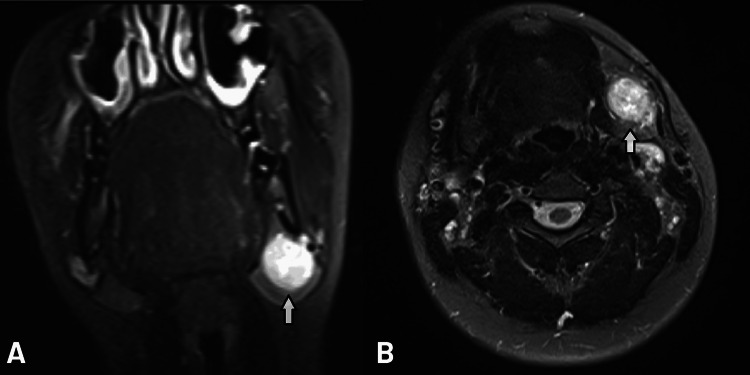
Preoperative magnetic resonance imaging (MRI) of the submandibular mass A: Coronal T2-weighted image shows the craniocaudal extent of the mass (arrow) and its anatomical relationship with the submandibular gland parenchyma and adjacent soft tissues. The predominantly hyperintense signal further supports the presence of cystic components. B: Axial T2-weighted image demonstrates a well-defined, hyperintense mass (arrow) in the left submandibular region. The lesion exhibits heterogeneous signal intensity, with internal areas of high signal suggestive of cystic degeneration and foci of low signal consistent with dystrophic calcifications.
